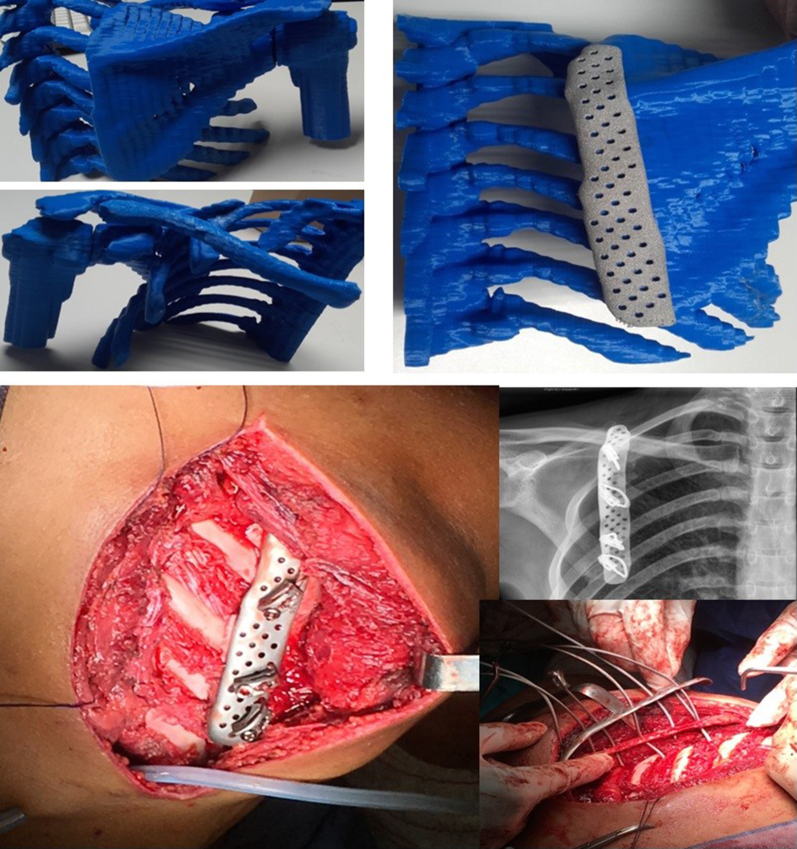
Preoperative model and intraoperative imaging of a winged scapula prosthesis and postoperative radiograph.
